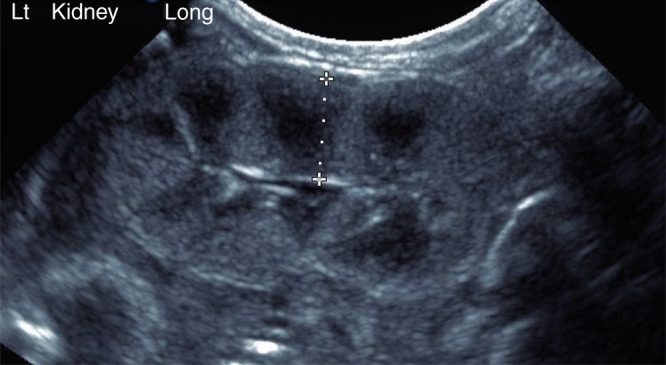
Neonatal kidney ultrasound. Sagittal ultrasound image of a preterm infant’s kidney at 32-weeks postmenstrual age with measurement of the renal parenchymal thickness of 7.2 mm.
